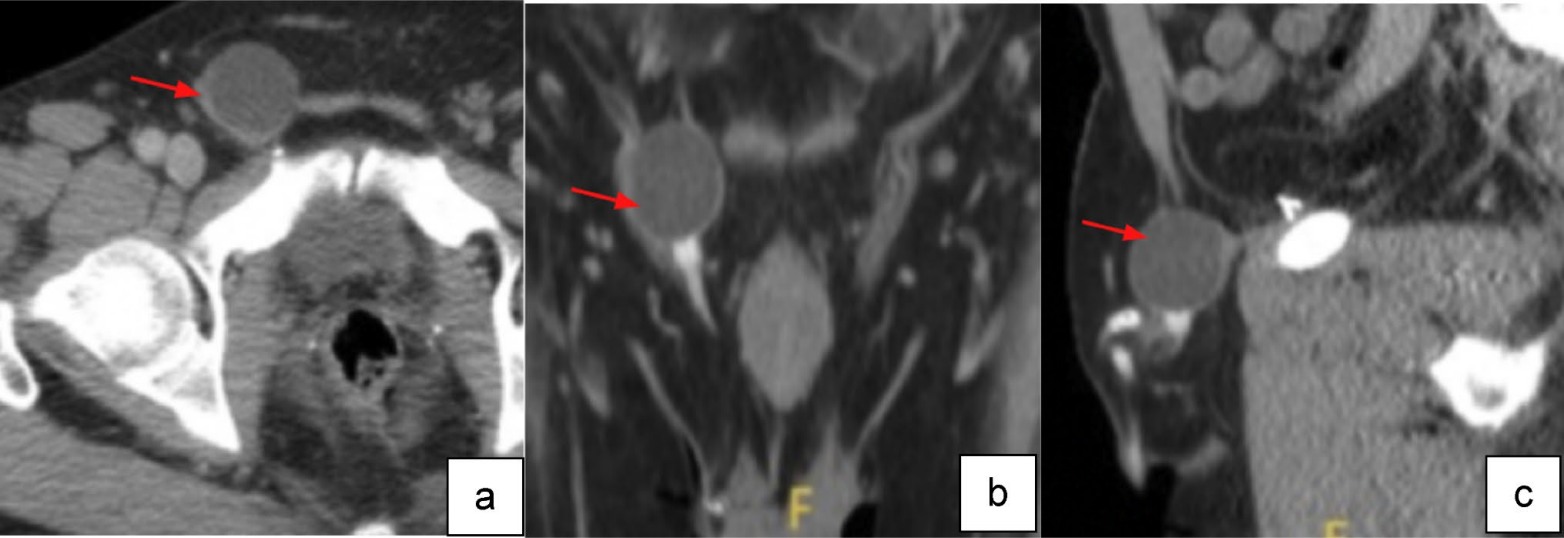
Axial (a), coronal (b) and sagittal (c) CT images with IV contrast show reservoir of IPP in the inguinal canal (red arrows)
